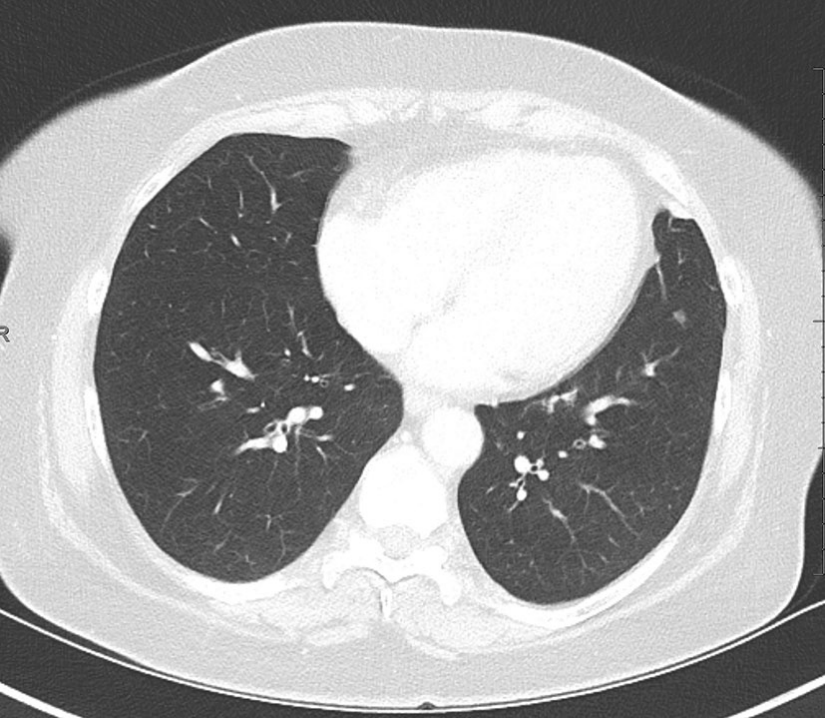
Axial CT image shows a 5 mm partially solid nodule in the left lower lobe that was in follow-up in a patient with a previous renal cell carcinoma. No other remarkable findings were found in the chest CT scan. This exam was categorized as CO-RADS 1, because the finding was clearly non-infectious
